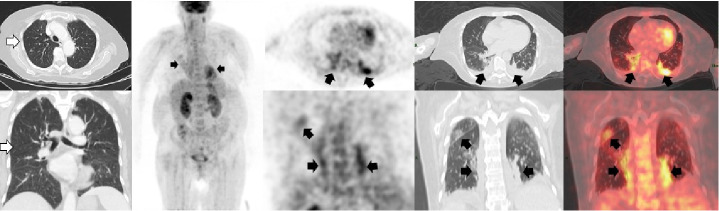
Tomografía computarizada de alta resolución de tórax (flechas blancas) en la que se observaron cambios fibroatelectásicos y bronquiectasias por tracción en el lóbulo superior derecho. En la PET/CT F18-FDG (flechas negras) no hubo evidencia de lesiones hipermetabólicas sospechosas de compromiso secundario. Sin embargo, en el tórax se observaron múltiples opacidades con patrón de vidrio esmerilado y aumento difuso del trazador y SUVmáx de 4, localizadas en ambos campos pulmonares con una consolidación hipermetabólica del segmento posterior del lóbulo inferior izquierdo y derrame pleural bilateral sin actividad metabólica.
